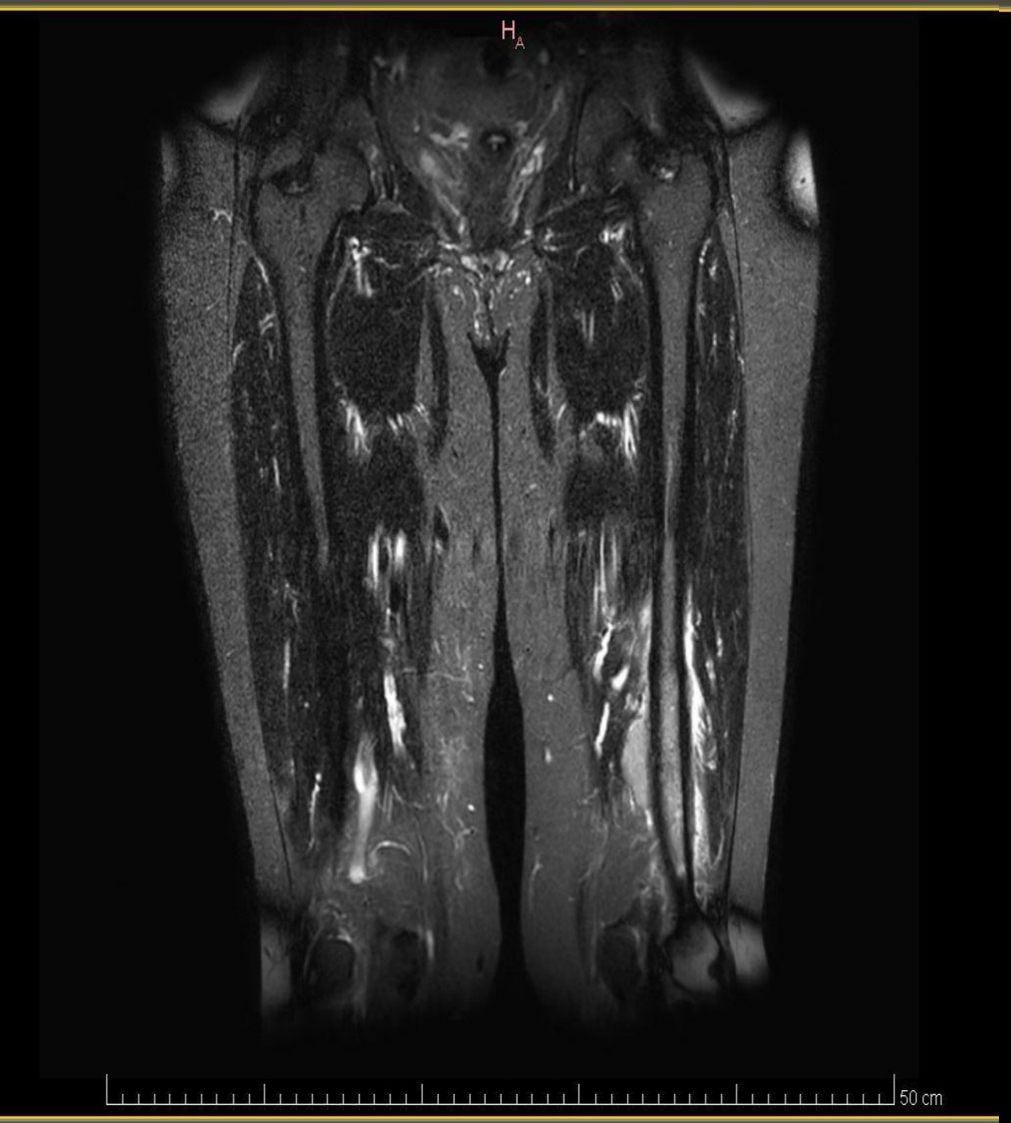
MRI of the legs showing a well-defined lobulated lesion on the left distal femur (arrow), suspicious for plasmacytoma.
